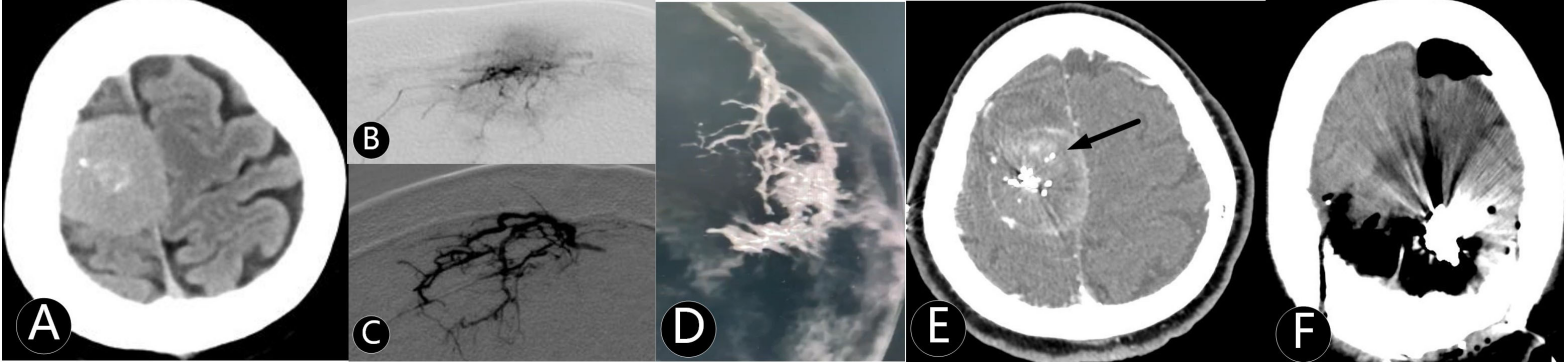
The patient was admitted several times for seizures. (A) CT showed right parasagittal mass with 75 cm^3^. (B) Microcatheter imaging showed the tumor outline. (C–E) The tumor was embolized subtotal by Onyx 18. (F) The mass was completely removed at second day after embolization.
